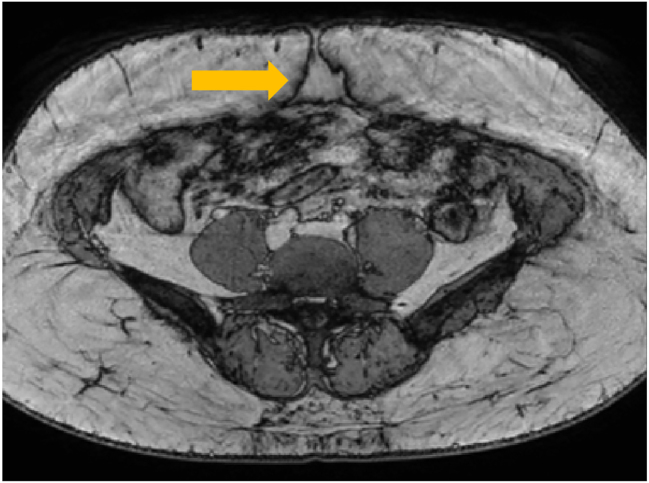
MRI of the abdominal wall showing a contrast-enhancing mass localized within the rectus muscle (yellow arrow), without diffusion restriction, aiding in preoperative characterization and surgical planning.
